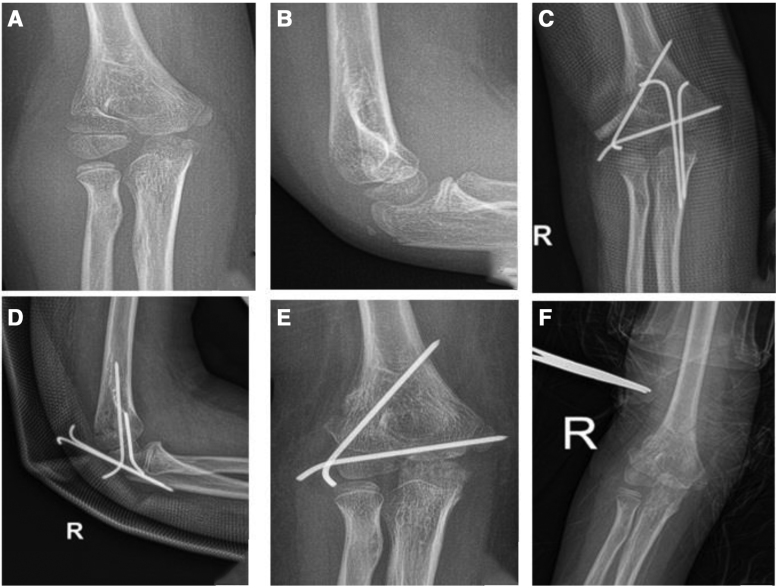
(A), (B) X-rays of a 9-year-old boy with a Song type 3 lateral condyle fracture and associated Mayo type I olecranon fracture. (C), (D) X-rays displayed with ORIF and CRPP. (E), (F) The removal results of K-wires. CRPP = closed reduction and percutaneous pinning, ORIF = open reduction and internal fixation.
